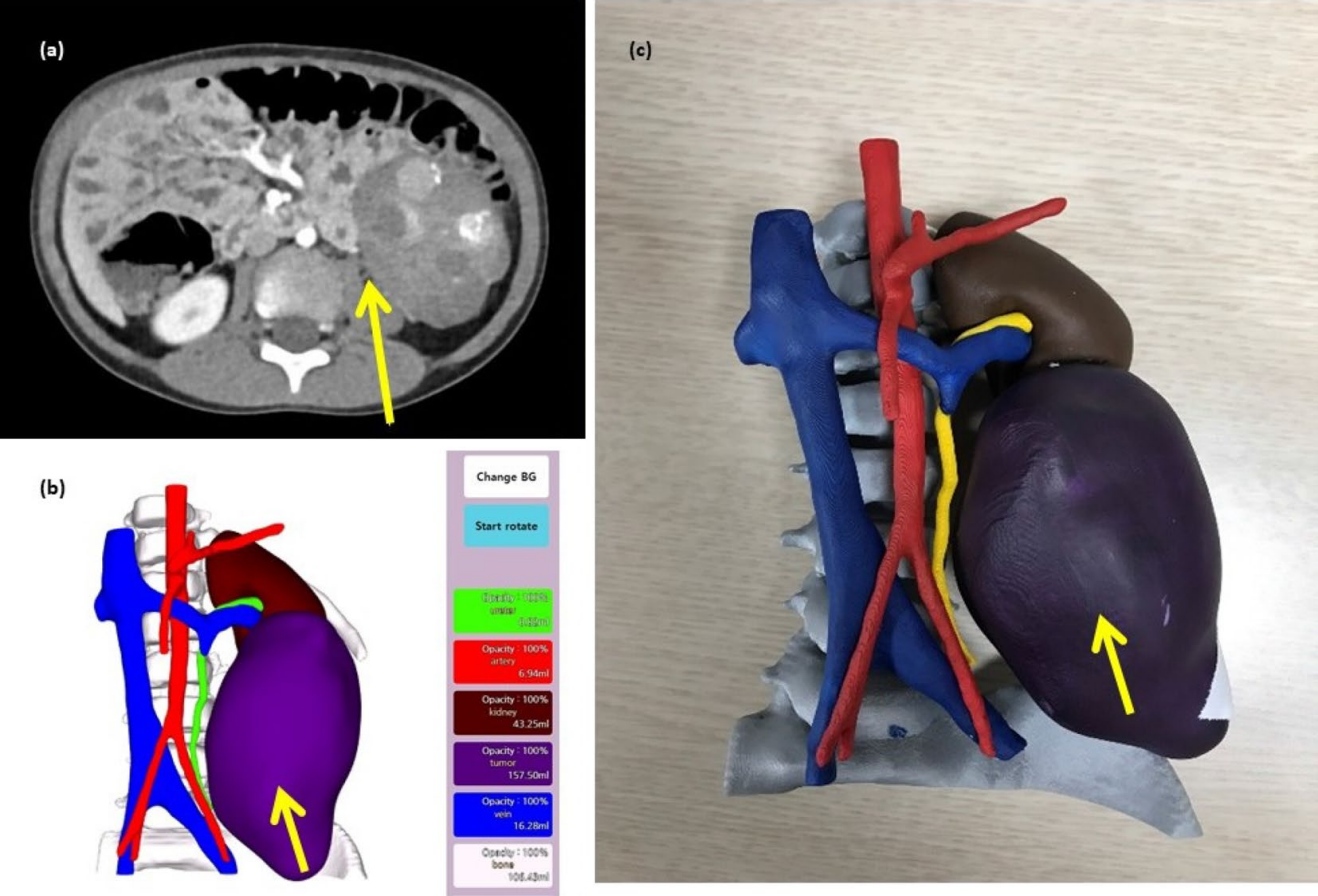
Computed tomography scan of a 4-year-old male patient with neuroblastoma (a), 3D reconstruction (b), and 3D printed model.
